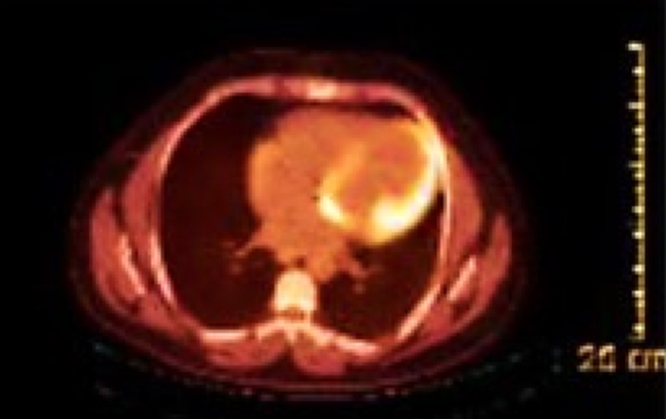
Positron Emission Tomography/Computed Tomography With Fluorodeoxyglucose Showing Increased Myocardial Uptake Consistent With Active Inflammatory Process (Maximum Standardized Uptake Value 6.6)
